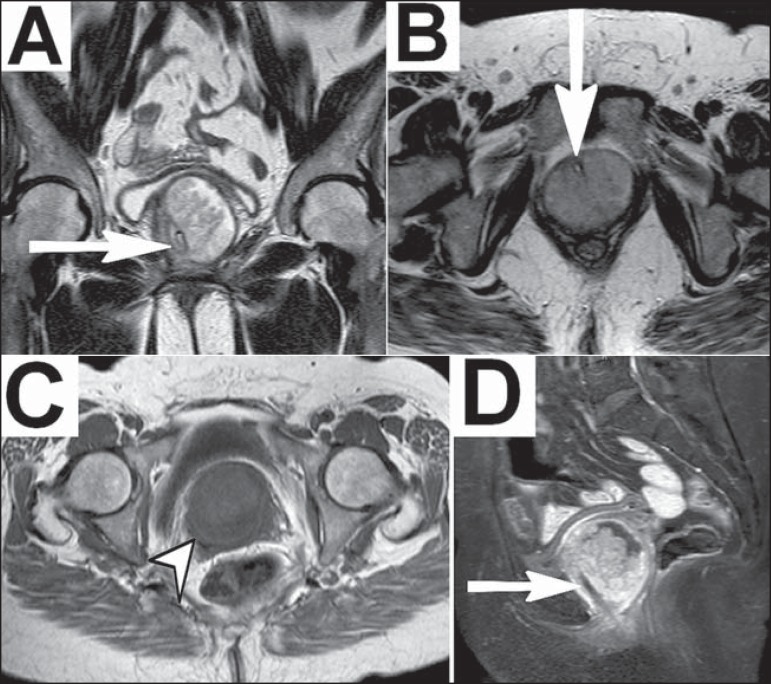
MRI of the pelvis. A,B: Coronal and axial T2-weighted sequences
